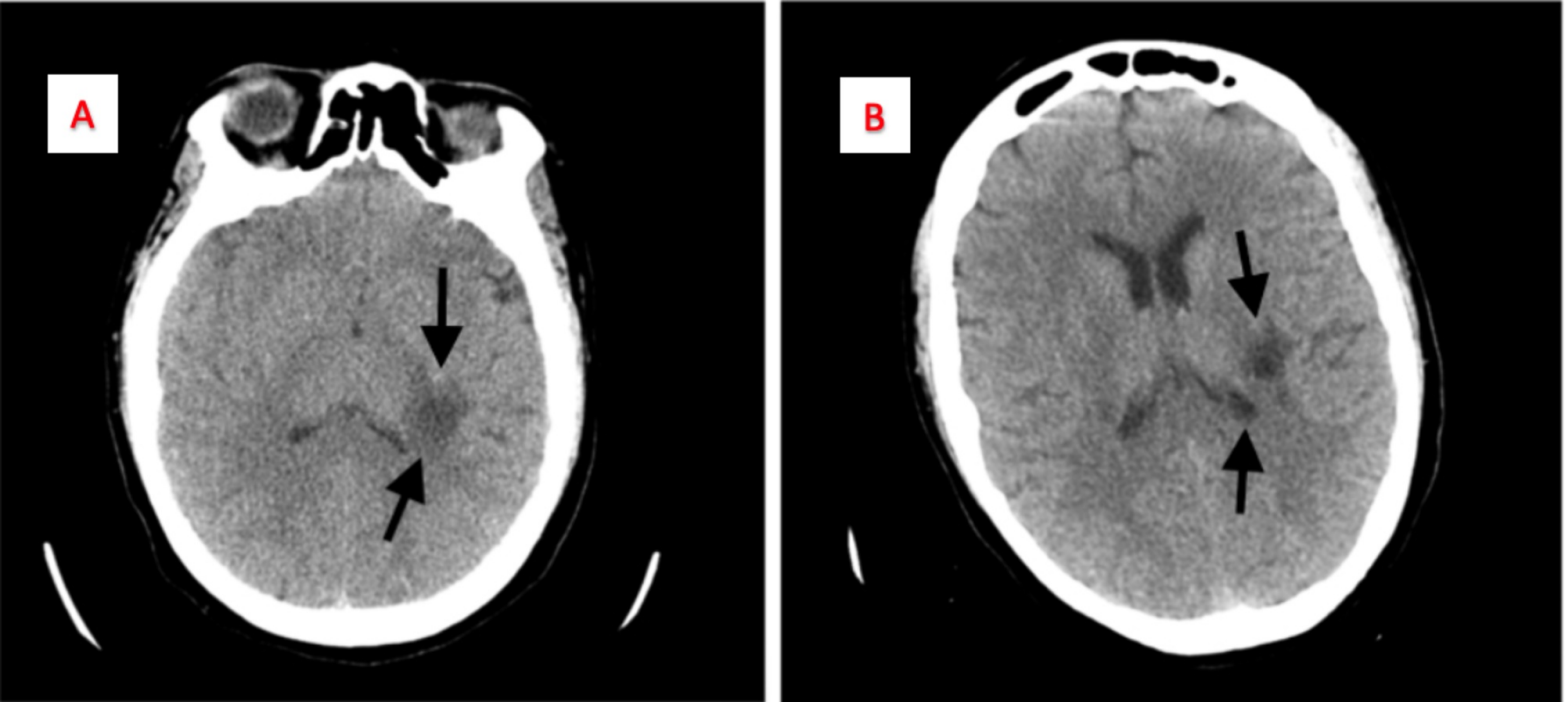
Computed tomography (CT) of the head without contrast Panel A: Displays a 2 x 1.5 cm hypodense lesion adjacent to the posterior basal ganglia/lateral thalamus. Panel B: Displays the same lesion at the level of the deep white matter adjacent to the posterior left lateral ventricle.
